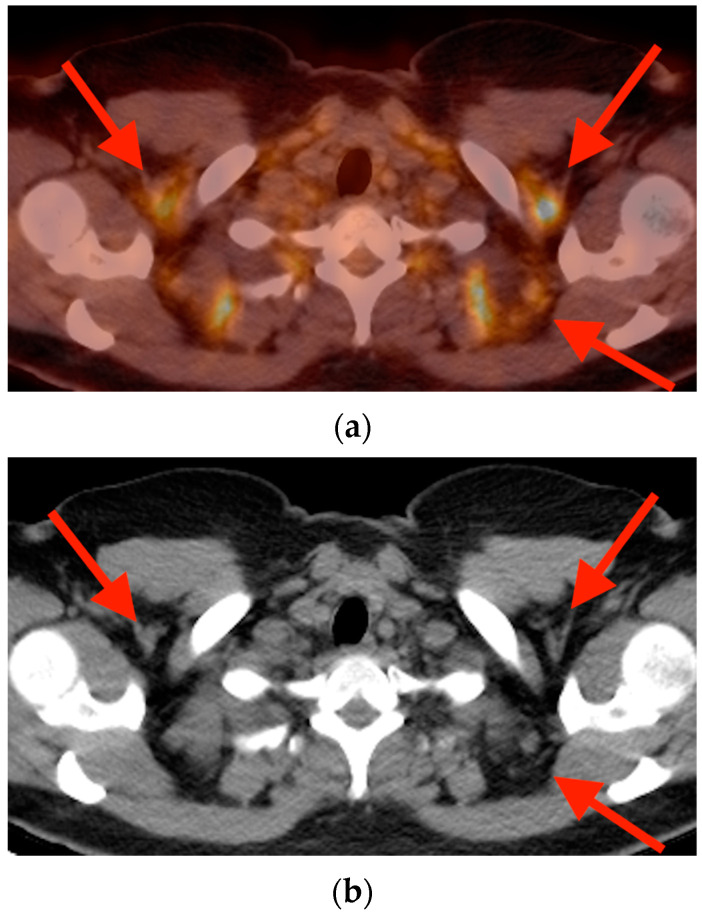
Axial FDG PET/CT fused (a) and non-fused CT (b) images obtained in a 41-year-old woman with cervical cancer demonstrate triangular-shaped areas of focal uptake in the cervical, supraclavicular, and paravertebral regions which correspond with low density areas of fat (red arrows), characteristic of brown fat activation. CT = computed tomography. FDG PET/CT = fluorine-18 fluorodeoxyglucose positron emission tomography/computed tomography.
